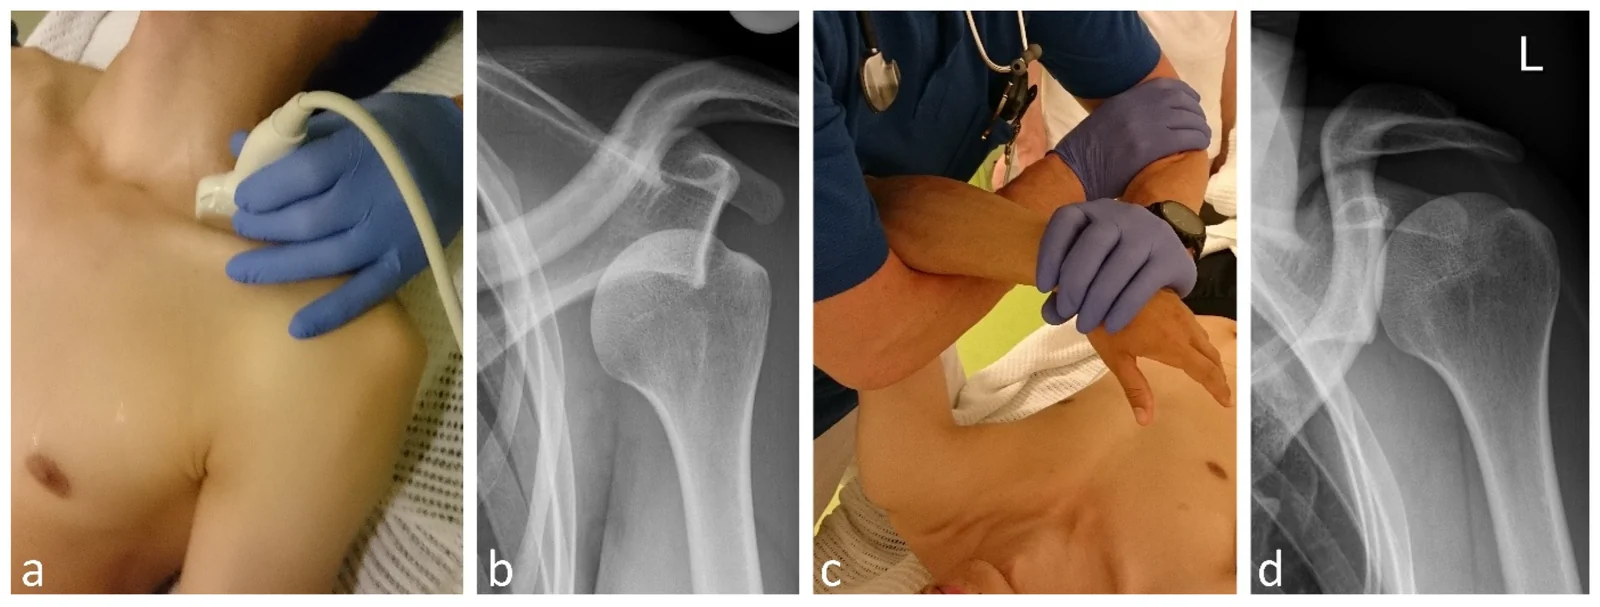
Clinical course of shoulder dislocation and reduction. (a) Clinical presentation of a patient with acute shoulder dislocation with ultrasound probe in the supraclavicular region. (b) Pre-reduction radiograph confirming anterior shoulder dislocation. (c) Reduction maneuver performed in the ED. (d) Post-reduction radiograph demonstrating successful joint re-alignment.
